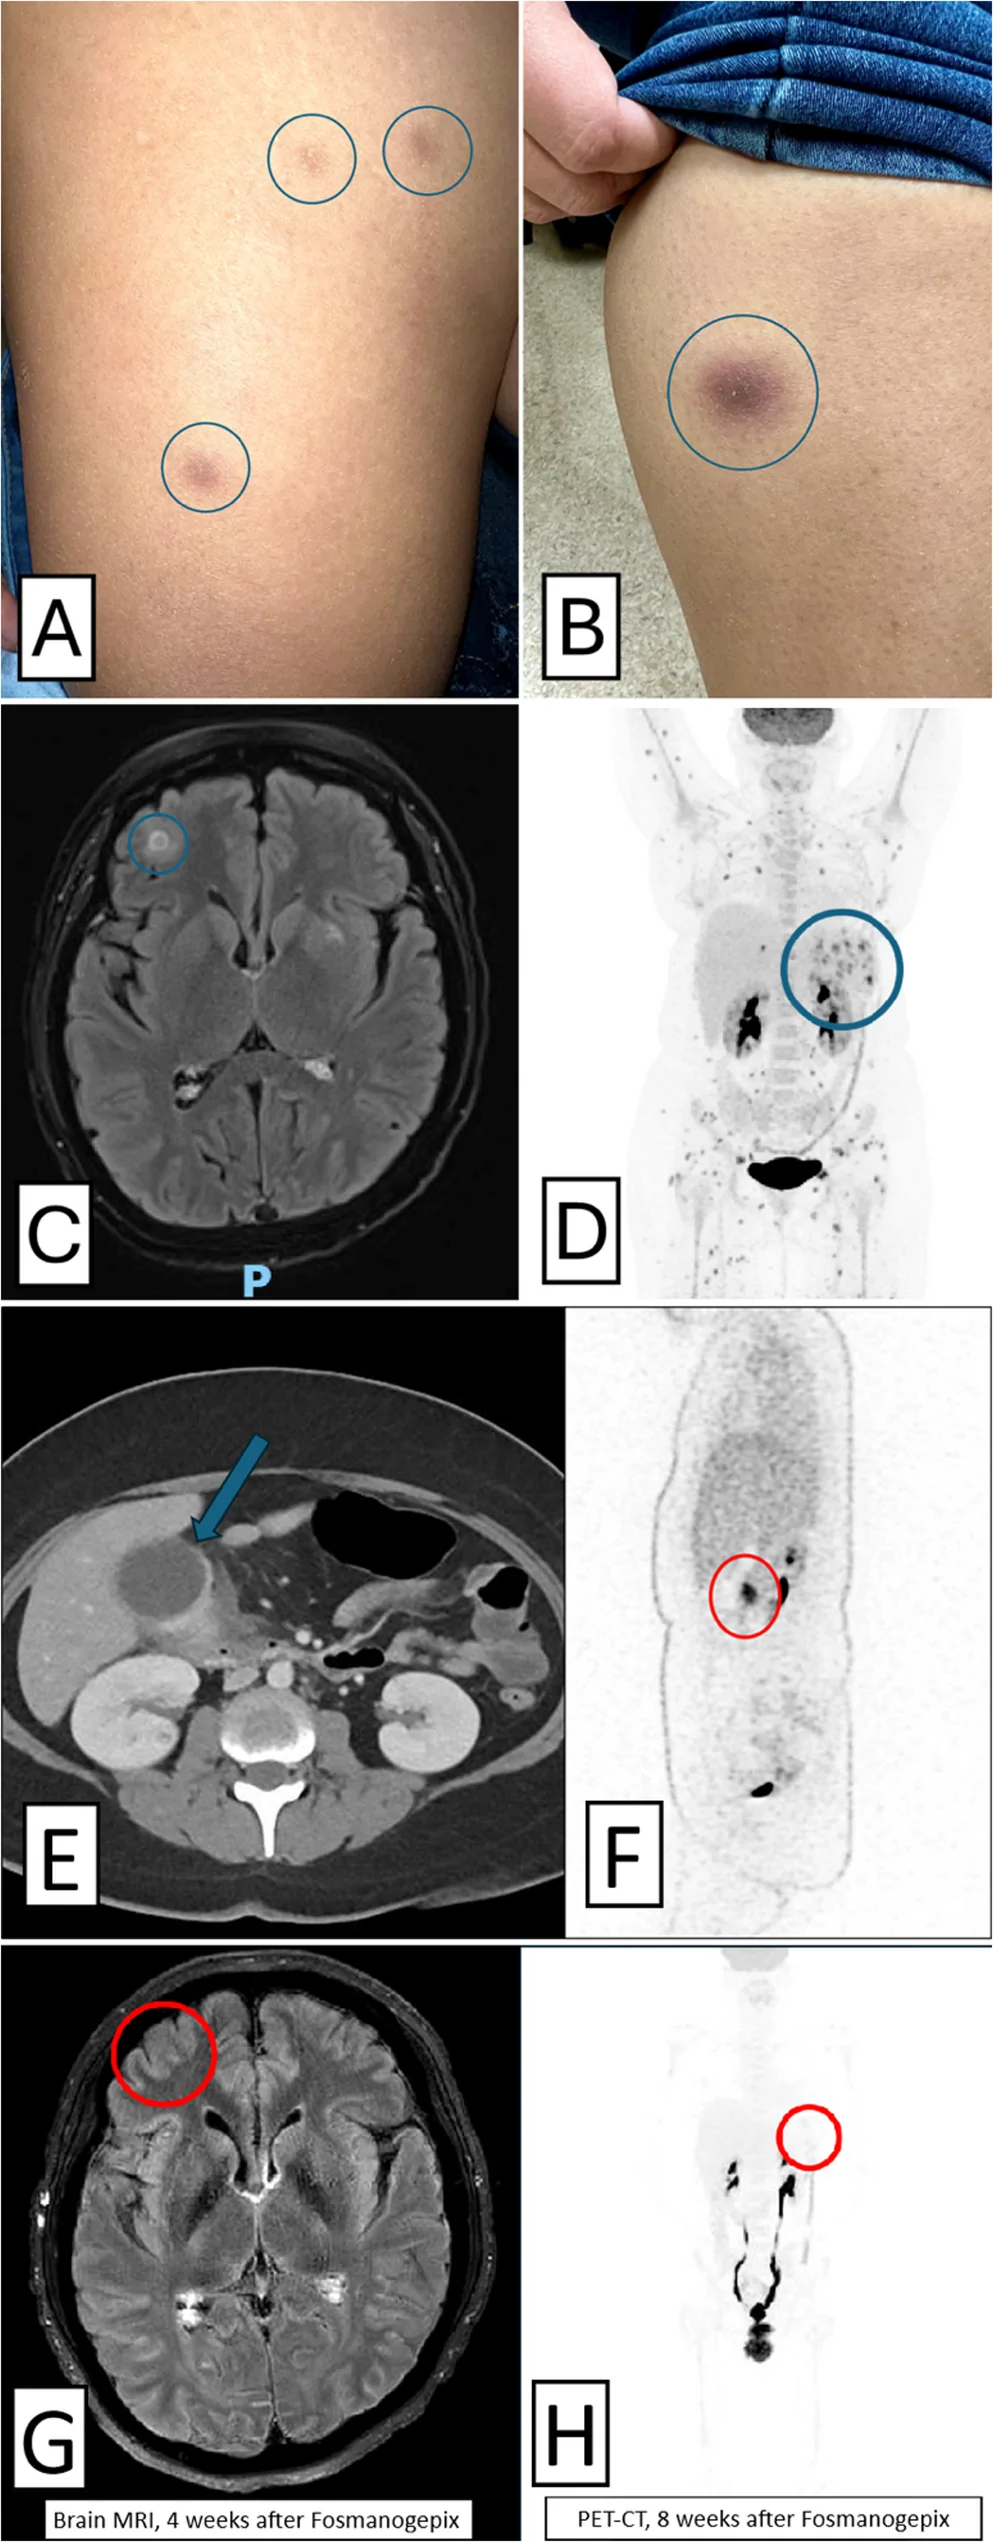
Exam findings on admission to NIH. 1A: Subcutaneous nodules noted on presentation to NIH, improved. 1B: New enlarging nodule on presentation, right thigh. 1C: Brain MRI (FLAIR) demonstrating a right frontal, ring-enhancing lesion (blue circle) and (1D) PET-CT noted disseminated lesions, with highlighted splenic nodules (blue circle). 1E: CT scan of the abdomen at the time of acute cholecystitis symptoms, demonstrating gallbladder wall thickening and inflammatory changes extending to the duodenum (blue arrow). 1F: PET-CT performed as part of surveillance just days prior to fever, which captures developing hyperintense signal at the gallbladder (red circle). 1G: Interval imaging with brain MRI 4 weeks after treatment notes resolution of previously seen ring-enhancing lesion (red circle). 1H: Repeat PET-CT after 8 weeks shows near complete resolution of disseminated hyperintense areas (red circle)
